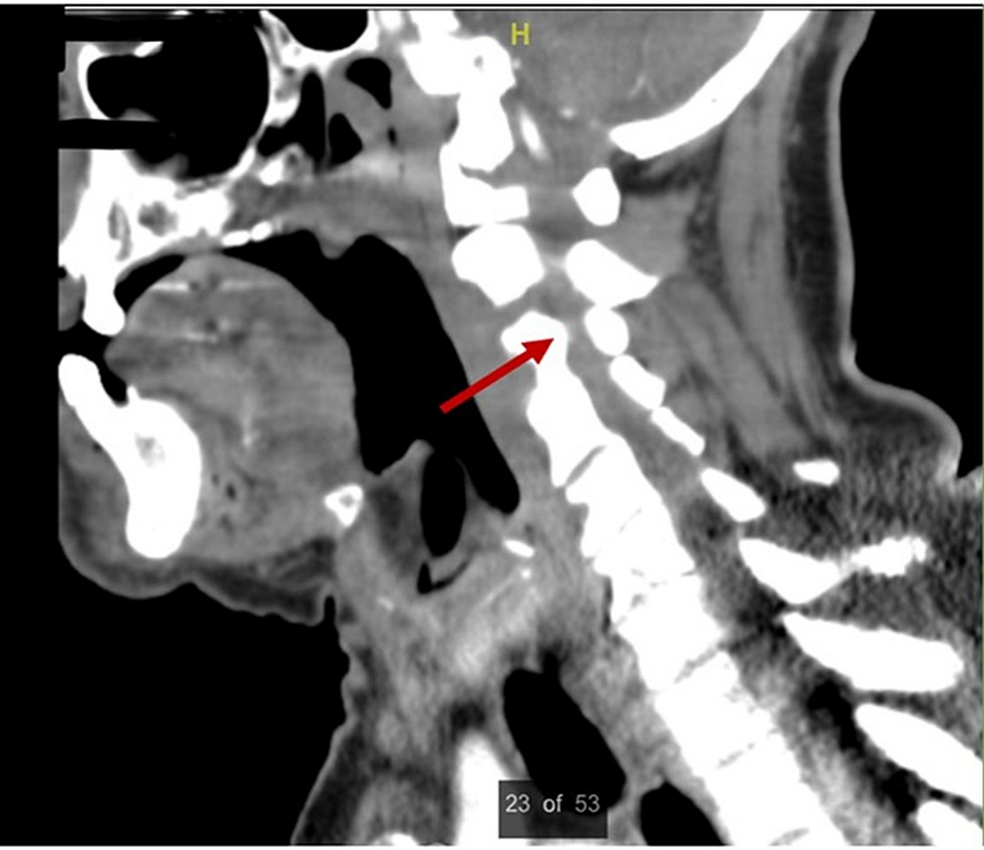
CT scan of the neck and spine. CT scan showing C3 cord compression, prominent diffuse sclerotic and lytic appearing osseous structures, and multilevel degenerative cervical spine changes.
